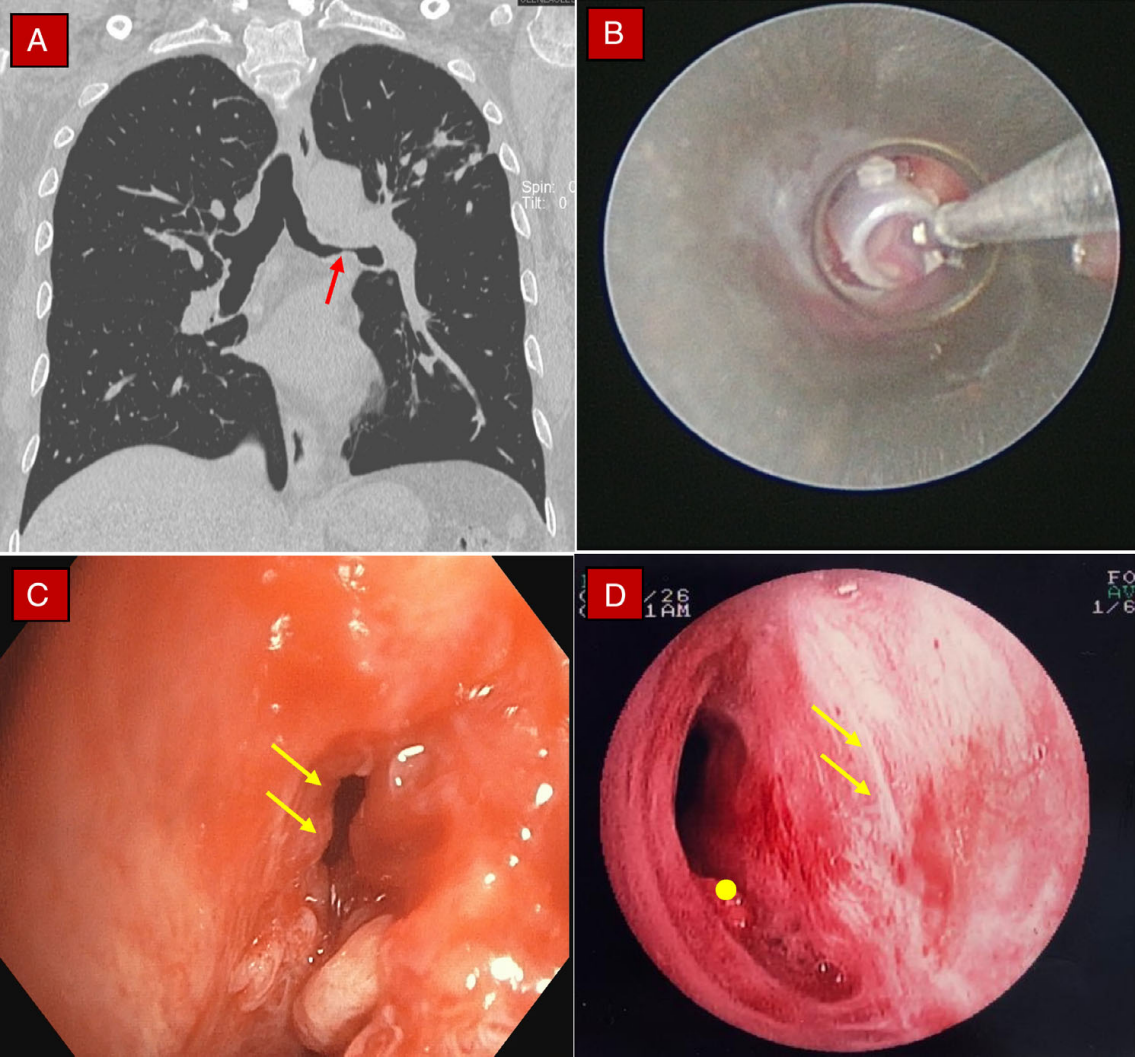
Computed tomography (CT) and serial bronchoscopy images. (A) CT demonstrating narrowing of the left main bronchus due to bronchomalacia (red arrow). (B) Adjusting the position of silicone stent deployed within the bronchomalacic segment by using grasping forceps inserted via rigid bronchoscope. (C) An approximately 2‐cm airway tear at the medial wall of bronchomalacic segment (yellow arrows). (D) Repeated flexible bronchoscopy one month later showing complete healing of bronchial tear (yellow arrows); persistent bronchomalacia marked with yellow circle.
